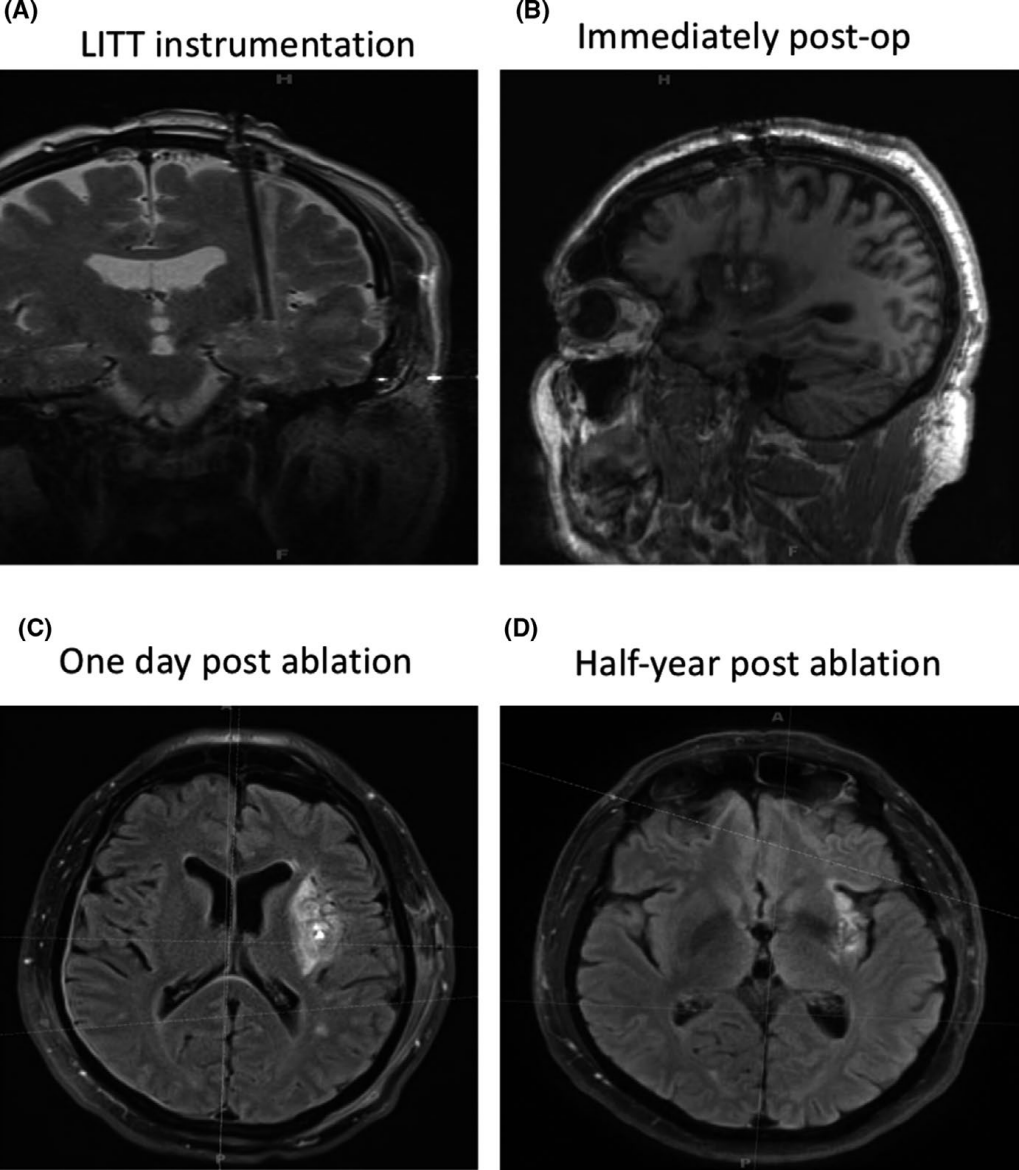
Brain MRI follow‐up of the patient after ablation. (A) LITT instrumentation shown by coronal image of brain MRI. (B) Brain MRI manifestation immediately postablation on sagittal image. Brain MRI manifestations one day (C) and half‐year (D) postablation on axial image
